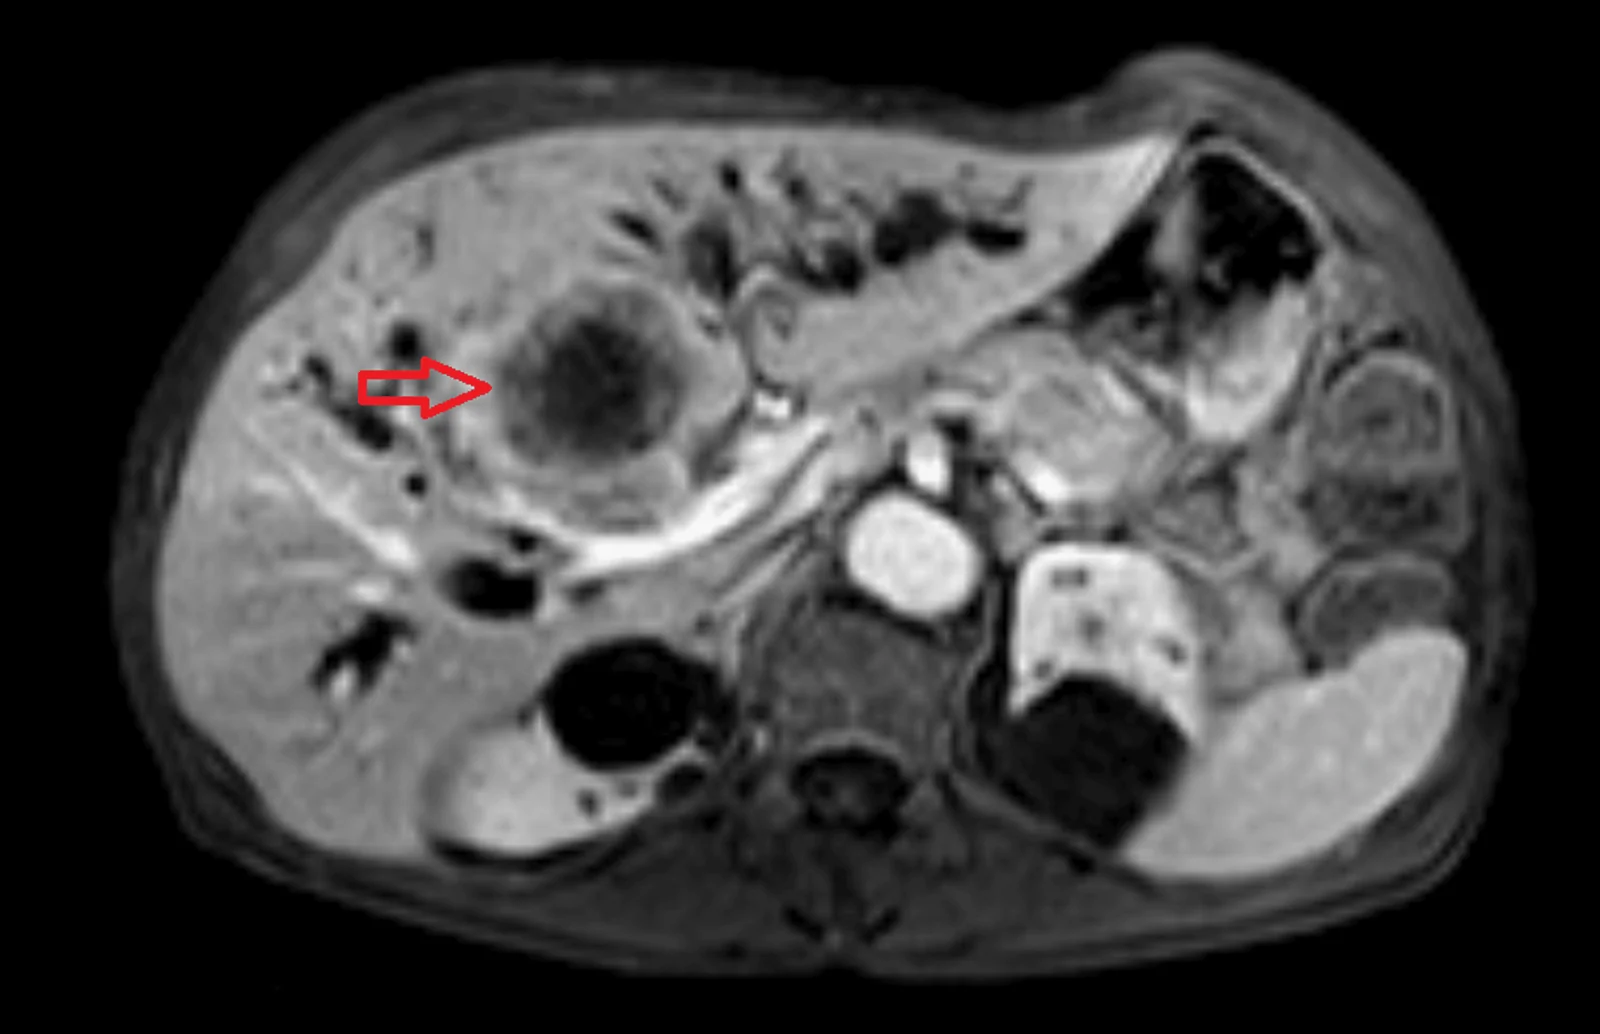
Axial contrast-enhanced T1-weighted MRI image demonstrating a large metastatic lesion in hepatic segment IV (red arrow). MRI: magnetic resonance imaging
